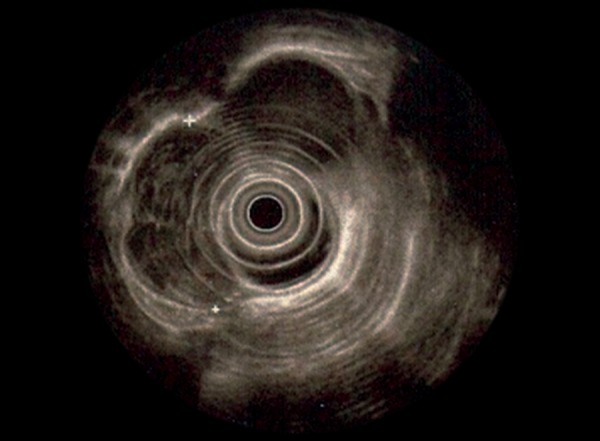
Endosonography showing a mass at distal esophagus 37.5 × 19.5 mm size, submucosally located, having smooth contours, heterogeneous hypoechoic and interpreted as compatible with mesenchymal tumor
